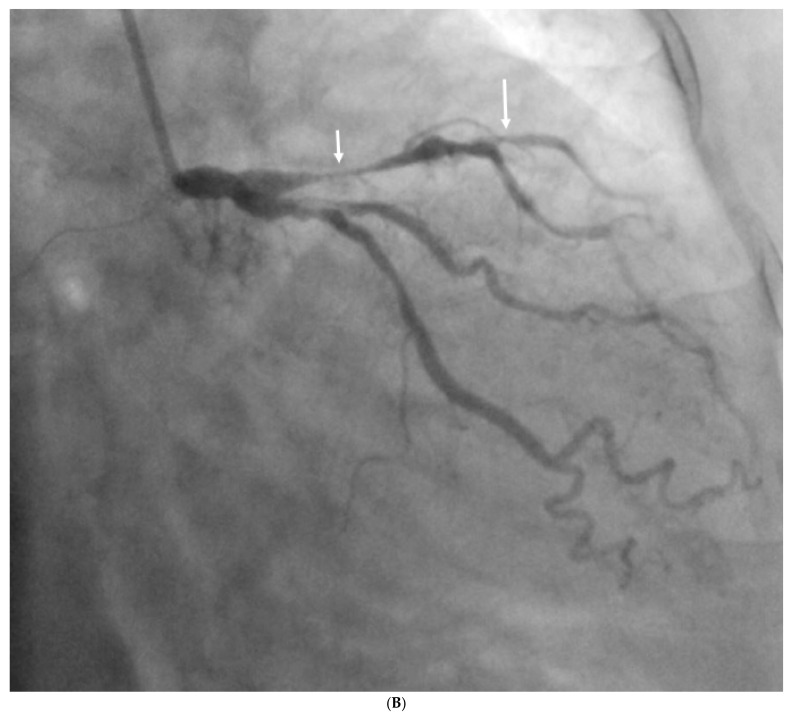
Multiple calcified plaques at the left anterior descending artery (LAD) in a 72-year-old female. Coronary stenoses were measured at 80%, 78%, 72%, and 70% corresponding to the original CCTA, Real-ESRGAN-HR, Real-ESRGAN-Average and Real-ESRGAN-Median images (short arrows in (A)), respectively. ICA (short arrow in (B)) confirms 75% stenosis. The distal stenoses at LAD due to calcified plaques were measured at 70%, 50%, and 51% stenosis on original CCTA, Real-ESRGAN-HR, and Real-ESRGAN-Average images but measured at 45% on Real-ESRGAN-Median images (long arrows in (A)). ICA confirmed the only 37% stenosis (long arrow in (B)). CCTA—coronary computed tomography angiography; ESRGAN—enhanced super-resolution generative adversarial network; HR—high resolution; ICA—invasive coronary angiography, Real-ESRGAN—real-enhanced super-resolution generative adversarial network. Reprinted with permission under open access from Sun and Ng [89].
